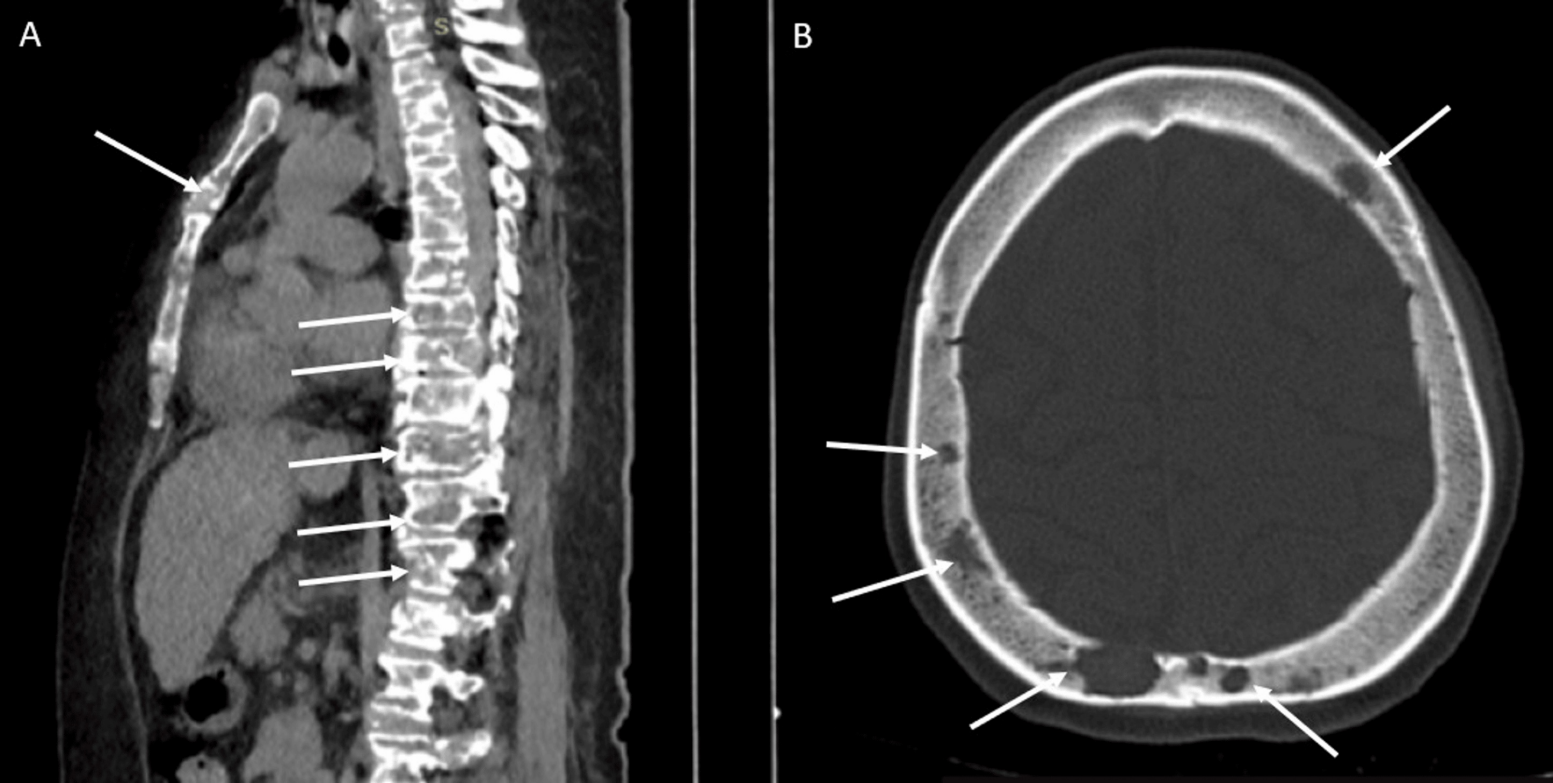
Computed Tomography (CT) Findings of Osteolytic Bone Lesions (A) Sagittal CT reconstruction showing multiple osteolytic lesions involving the vertebral bodies and sternum (white arrows). (B) Axial skull CT demonstrating multiple punched-out osteolytic lesions of the calvarium (white arrows).
